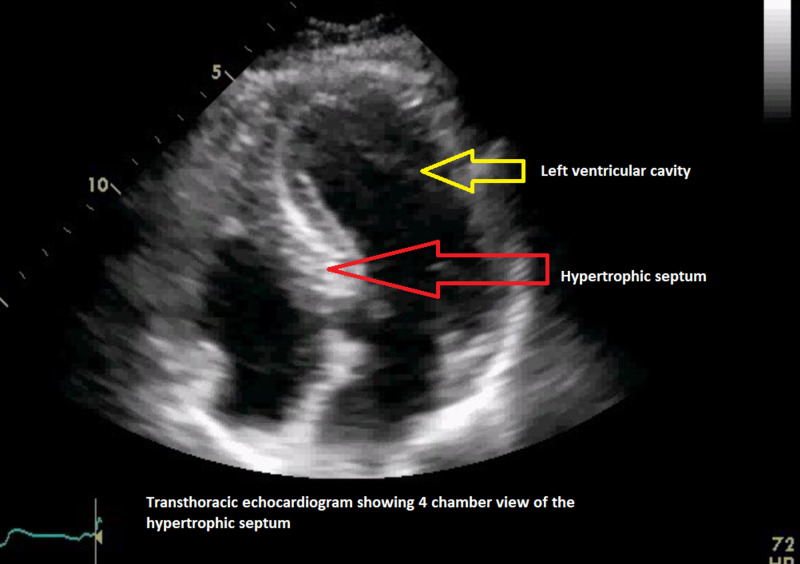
Transthoracic echocardiogram showing hypertrophic septum with a diastolic thickness of 2 cm (normal 0.6-1.1 cm).
